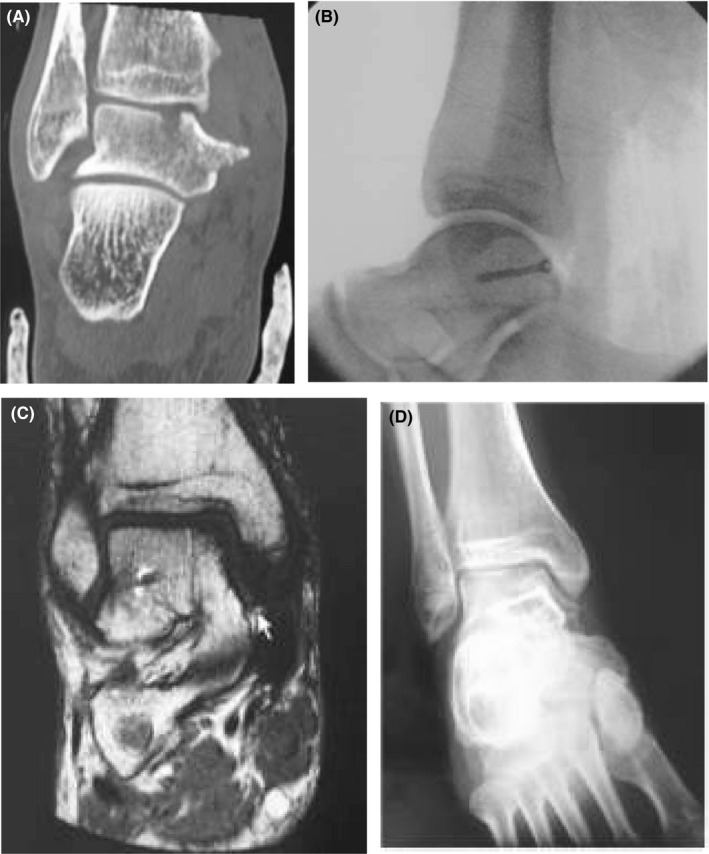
A, Vertical slice of the initial computed tomography scan showing the fracture of the posteromedial tubercle and the posteromedial talar dome which extends to the tibiotalar joint. B, Intraoperative radiograph of the open reduction and the internal fixation of the fracture with a 3.5 mm cancellous screw. C, MRI examination during the last follow‐up, 3 y postoperatively, showing the undisplaced cancellous screw in the talar body, in both slices. D, Radiograph during the last follow‐up, 3 y postoperatively, showing the undisplaced cancellous screw in the talar body, as well as the absence of post‐traumatic arthritis of the tibiotalar joint
